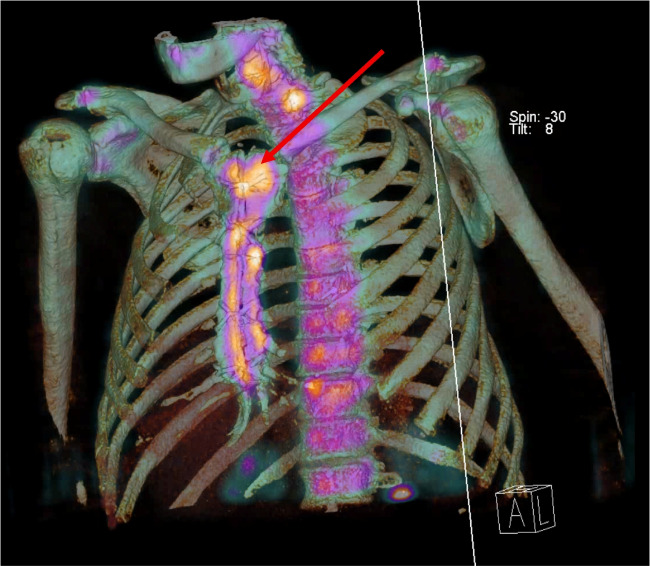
3D reformat of a SPECT CT demonstrating high signal within the sternum
